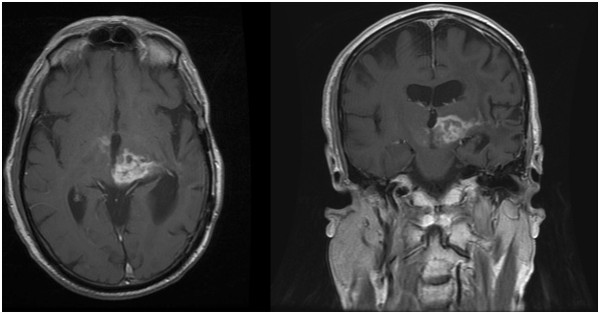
MRI findings on day 21 after sonication of the tumor. Axial (TR = 766
